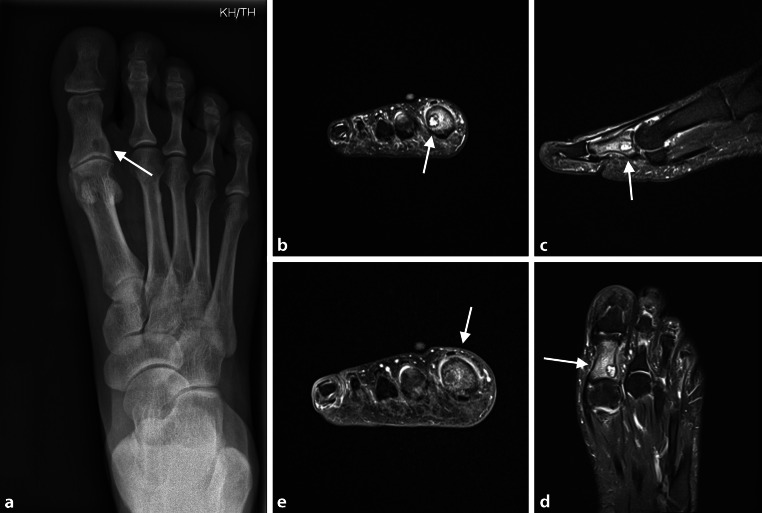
a 55-year-old female patient with osteoid osteoma of the 1st proximal phalanx of the right foot. X‑ray with lytic lesion and cortical thickening at the medial aspect of the base of the 1st proximal phalanx (*arrows* pointing at the regions of interest). b–e MRI scans showing well-circumscribed sclerotic lesion of 6 mm in size with extensive bone edema. Minor synovial reactive effusion at the 1st metatarsophalangeal joint (b T2 Turbo spin echo (TSE) fatsat (FS); c ,d T2 FS; e T1 TSE + Contrast Agent; arrows pointing at the regions of interest)
